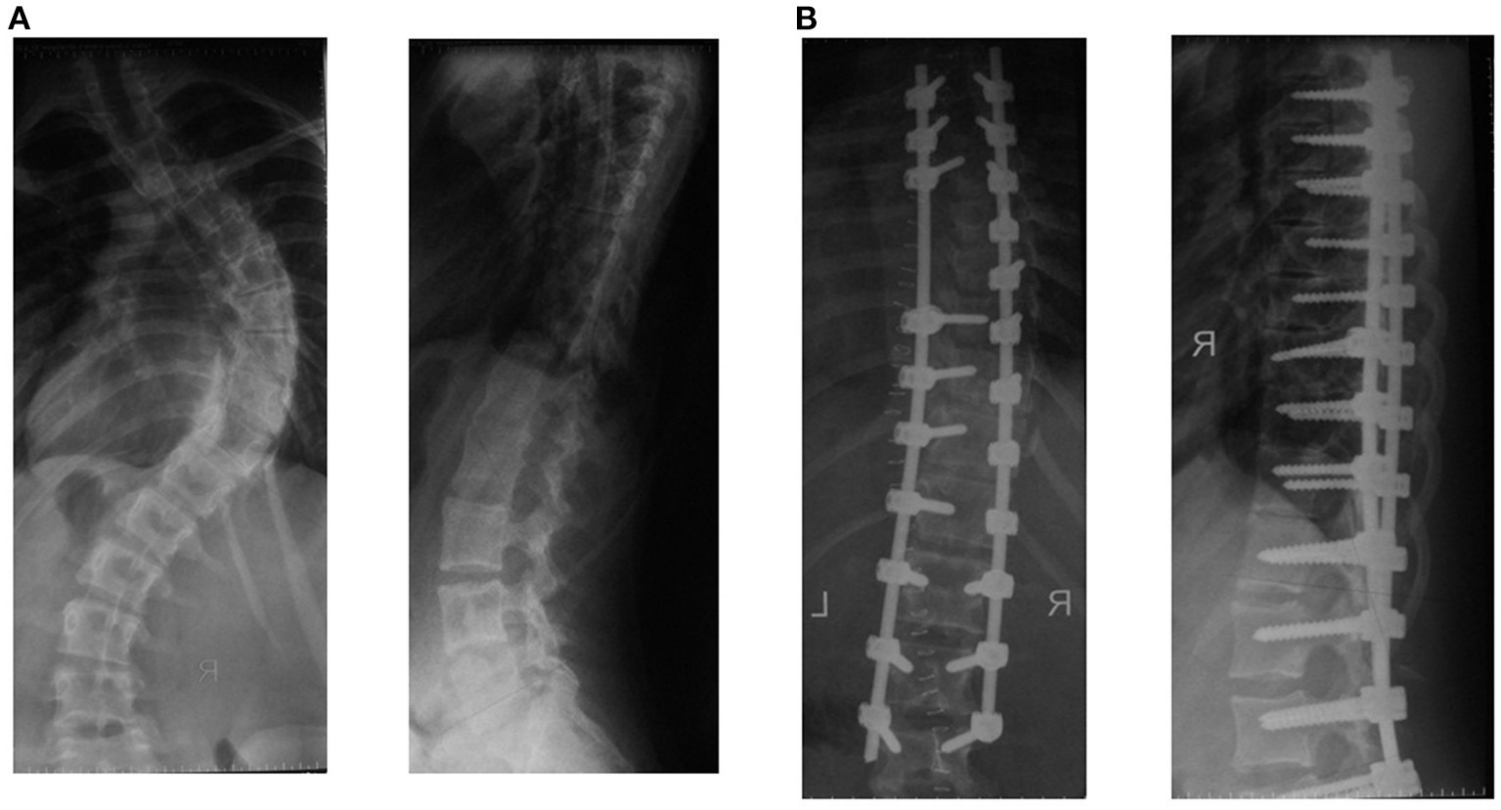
Spinal radiographs of patient B (16 years old). Idiopathic right thoracic scoliosis. (A) Preoperatively, the angle of deformity was 78° according to Cobb, the angle of kyphosis was 7°, and the angle of lordosis was 38°. (B) Postoperatively, the angle of scoliosis was 16° according to Cobb, the angle of kyphosis was 12°, and the angle of lordosis was 25°.
